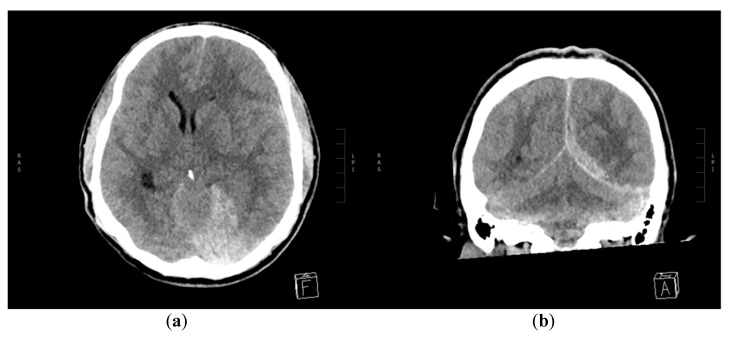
Control non-contrast CT of the brain upon readmission. (a) Axial and (b) coronal MPR reconstruction showing increased mass effect from the growing left acute subdural hematoma (aSDH) with midline shift to the right side, obliteration of quadrigeminal and both ambient cisterns, compression of the left lateral ventricle and dilatation of the right ventricular trigonum. The right-sided EDH is not shown.
